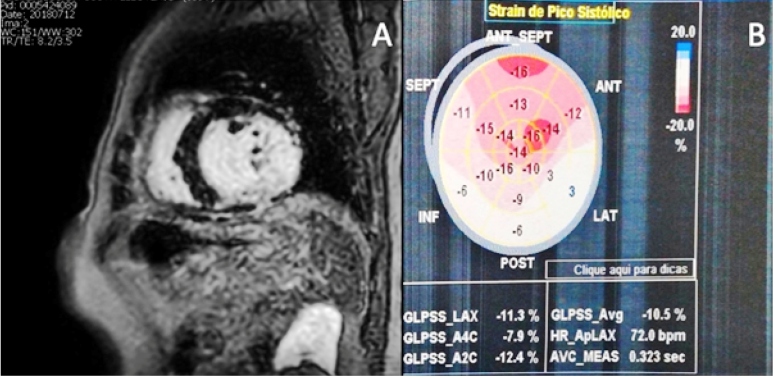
(A) Cardiac magnetic resonance imaging with the presence of late transmural enhancement in the lower-middle-basal wall; (B) Transthoracic echocardiogram with global longitudinal strain (-10.5%).
